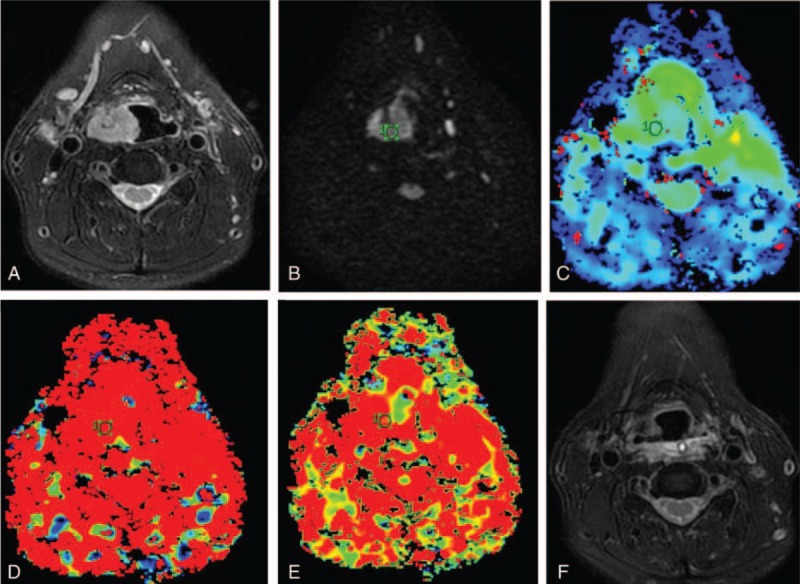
A 65-year-old man with right pyriform sinus carcinoma with good response to induction chemotherapy. (A) Transverse T_2_-weighted imaging. (B) Transverse diffusion weighted imaging (DWI) map obtained with *b* = 800 s/mm^2^. (C–E) The mean pretreatment *D*^∗^, *D*, and *f* values were 48.35 × 10^–3^ mm^2^/s, 0.775 × 10^–3^ mm^2^/s and 53.44% in responder, respectively. (F) Transverse T_2_-weighted imaging show that the mass disappeared completely after 2 cycle induction chemotherapy.DWI =  diffusion weighted imaging.
